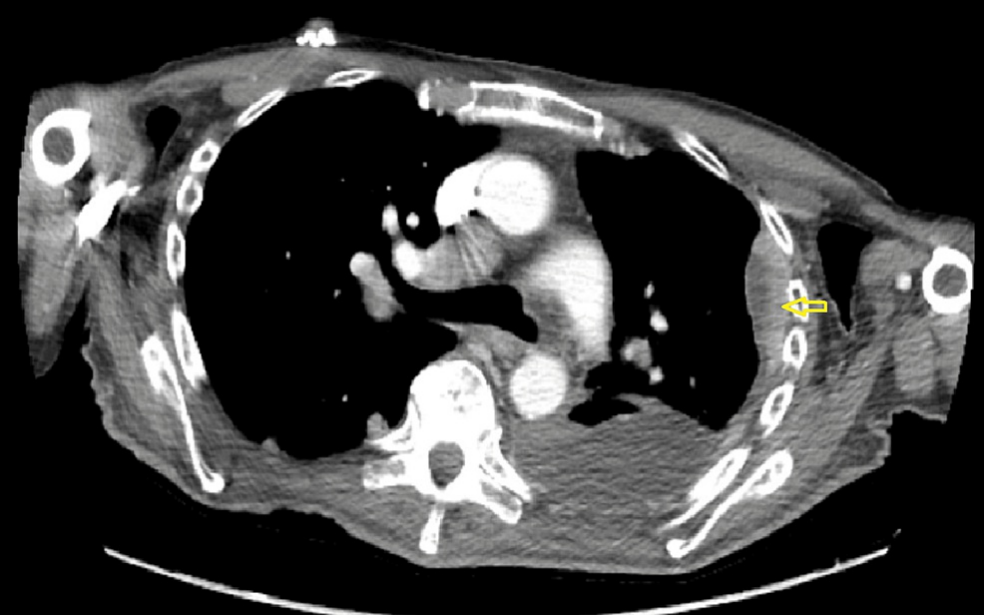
Chest CT demonstrating nodular pleural thickening in the left lung
